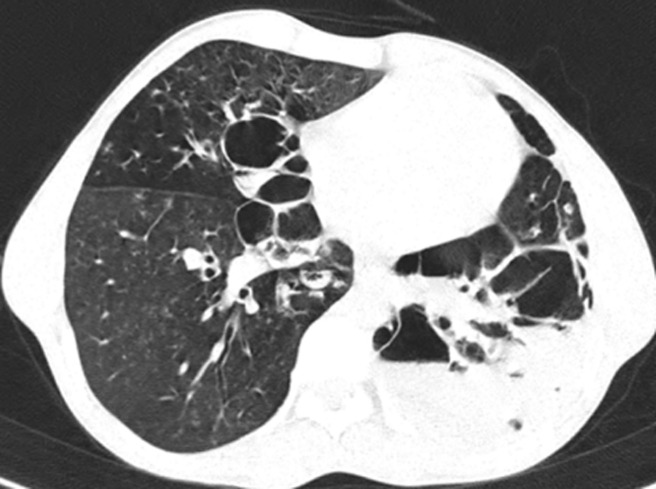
computed tomography of the chest showing bilateral variable-sized cystic and tractional bronchiectatic changes
